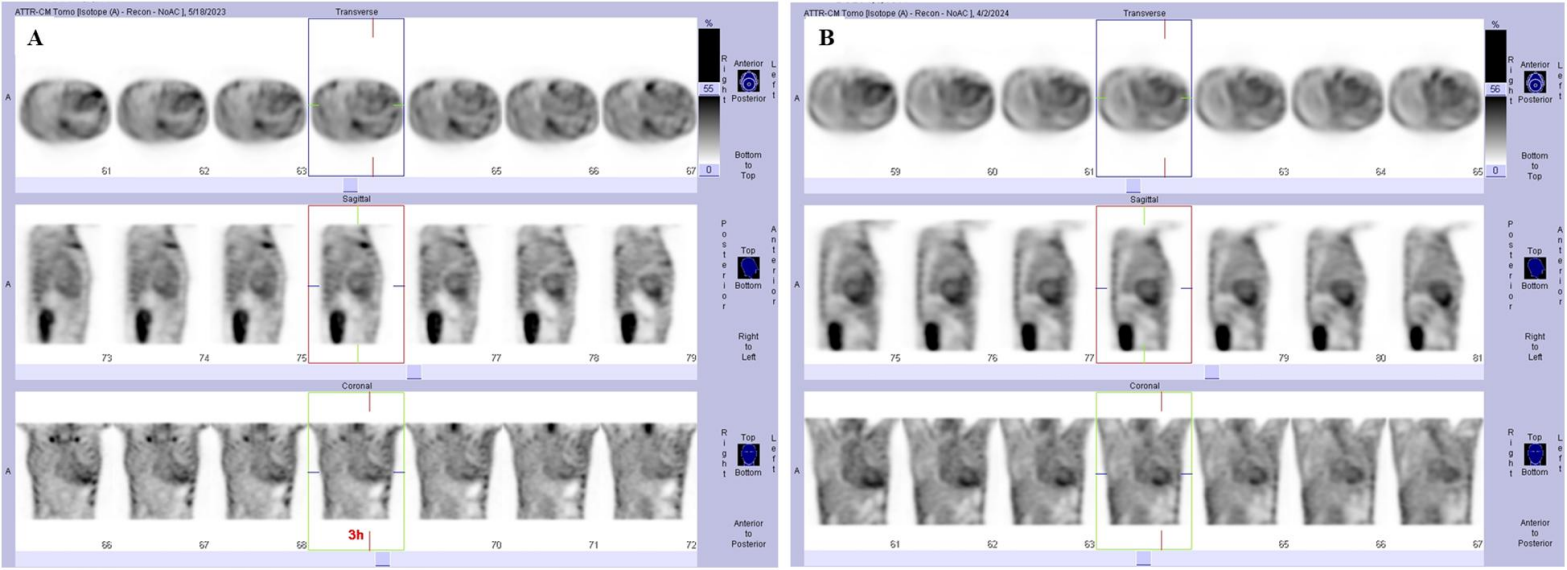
Nuclear ^99m^Tc-pyrophosphate scintigraphy images. (A) The imaging was graded visually as grade 2 uptake, with a heart/contralateral lung (H/CL) ratio of 1.27. (B) The imaging was interpreted as grade 3 uptake, with a H/CL ratio of 1.35.
